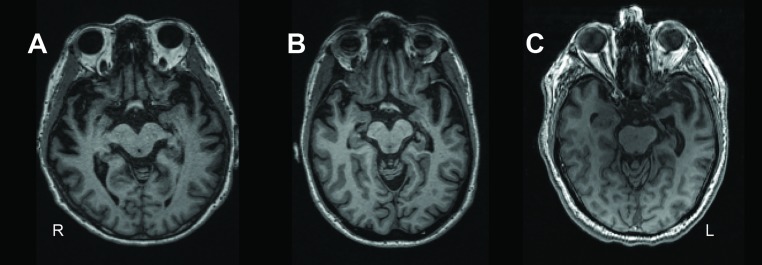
Temporal pole atrophy in patients. Transversal T1-weighted MR images of patients representative for the distribution of dominant anterior temporal pole atrophy within the sample. From left to right: patient with lower quartile (A), median (B) and upper quartile (C) anterior temporal pole grey matter volumes of the dominant side.
